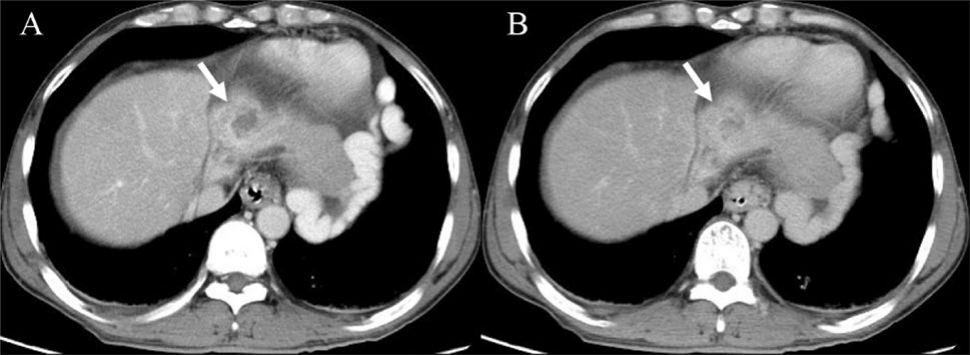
A case of sclerosing cavernous hemangioma mimicking intrahepatic cholangiocarcinoma (male, 51-years-old). Axial CT in the portal venous phase showed an irregular peripheral heterogeneous enhancement mass with capsular retraction, left lobe atrophy and mild biliary ductal dilatation in the left lobe (A), internal non-enhancement in the delayed phase (B) (arrows)
